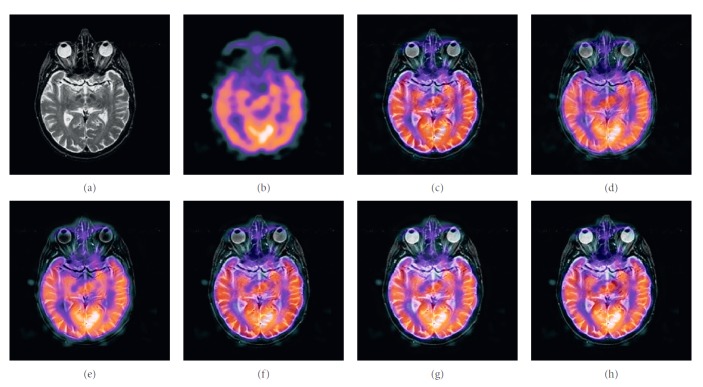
MR-T2/SPECT medical image fusion results. (a) MR-T2 original image. (b) SPECT original image. (c) Method 1. (d) Method 2. (e) Method 3. (f) Method 4. (g) Method 5. (h) FFST-SR-PCNN.
